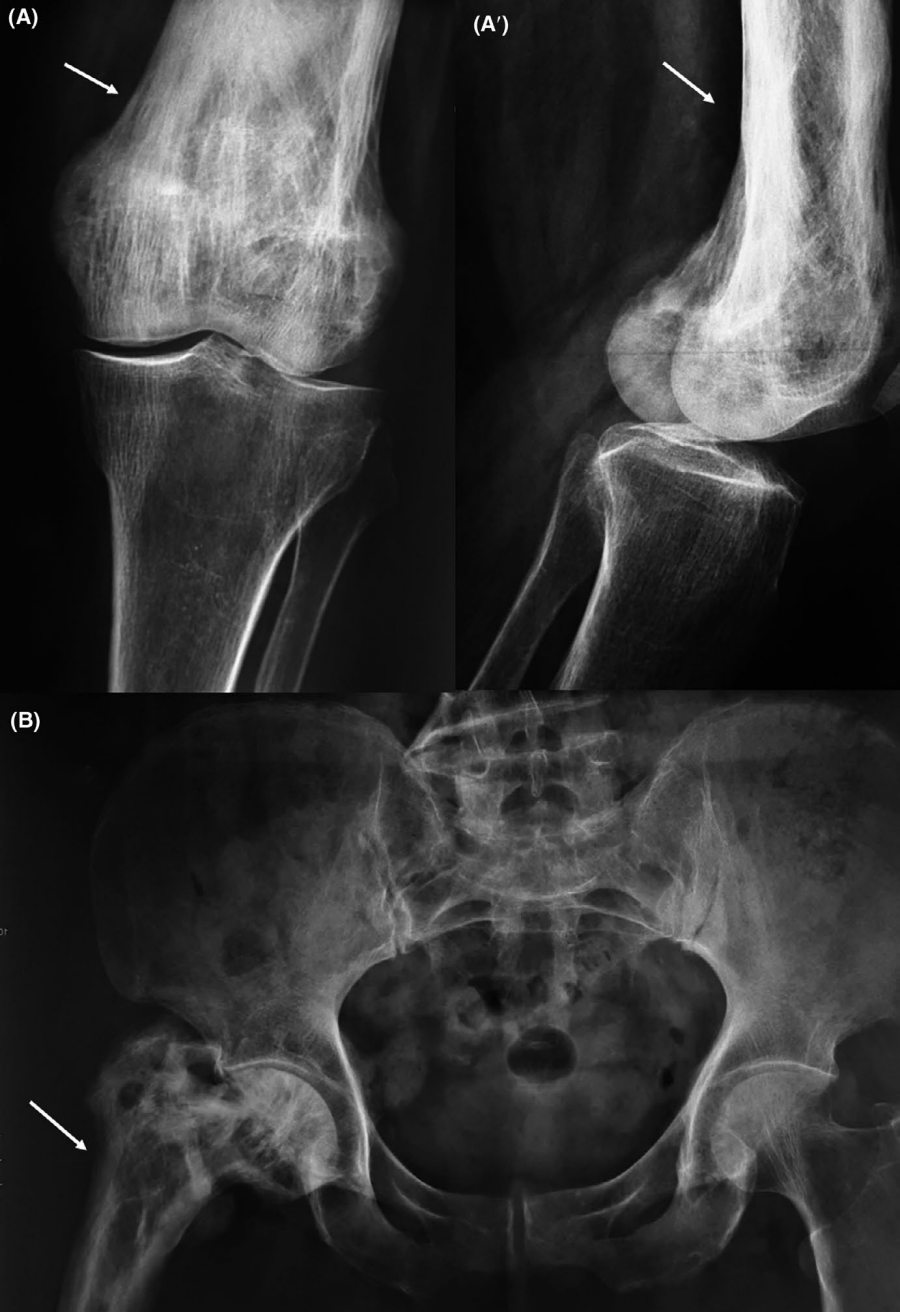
(A) Knee’s radiographs (anteroposterior view (A) and lateral view (A’) showing bony expansion with cortical thickening and loss of corticomedullary differentiation of the lower extremity of the left femur. (B) Pelvic radiograph showing a mixed appearance, including osteolytic and osteoblastic lesions, bone expansion and deformity of the left femur.
